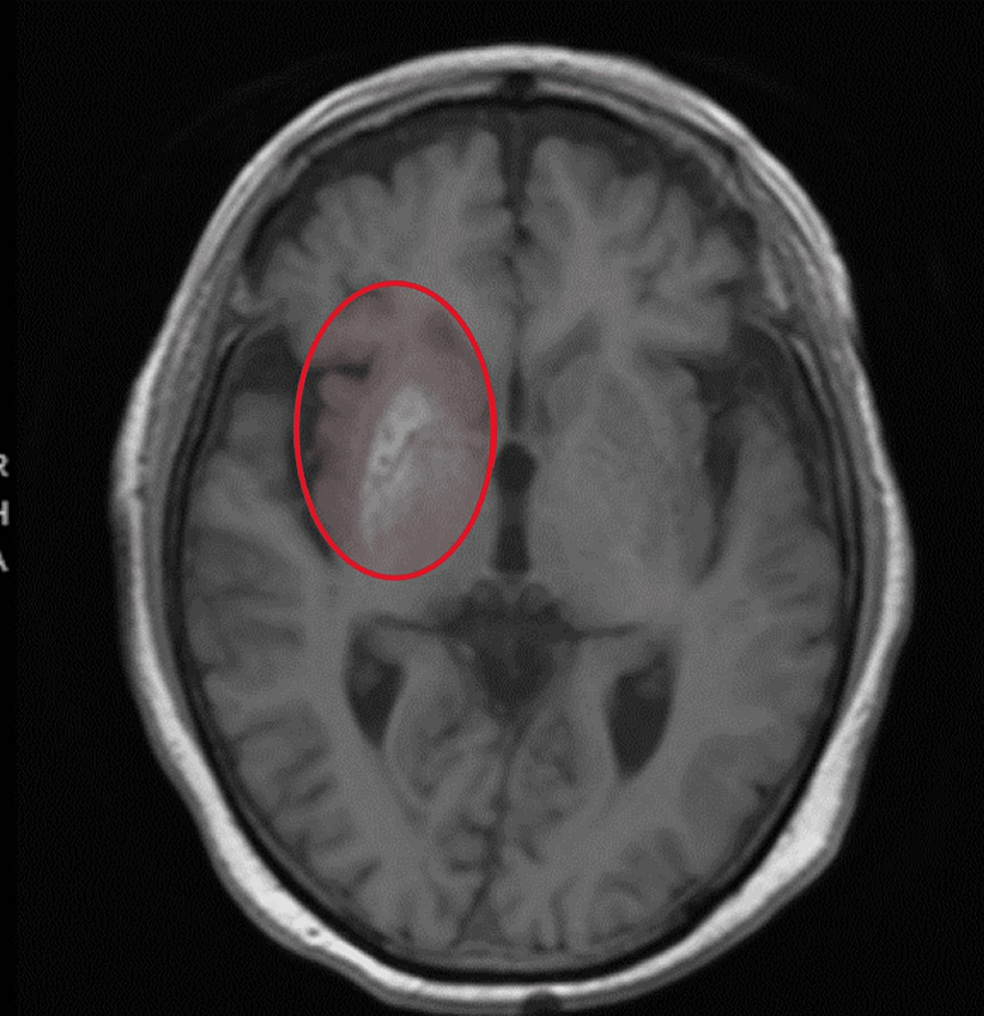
MRI T1 signal abnormality of the right basal ganglia (red oval). All other sequences were negative. MRI: magnetic resonance imaging
